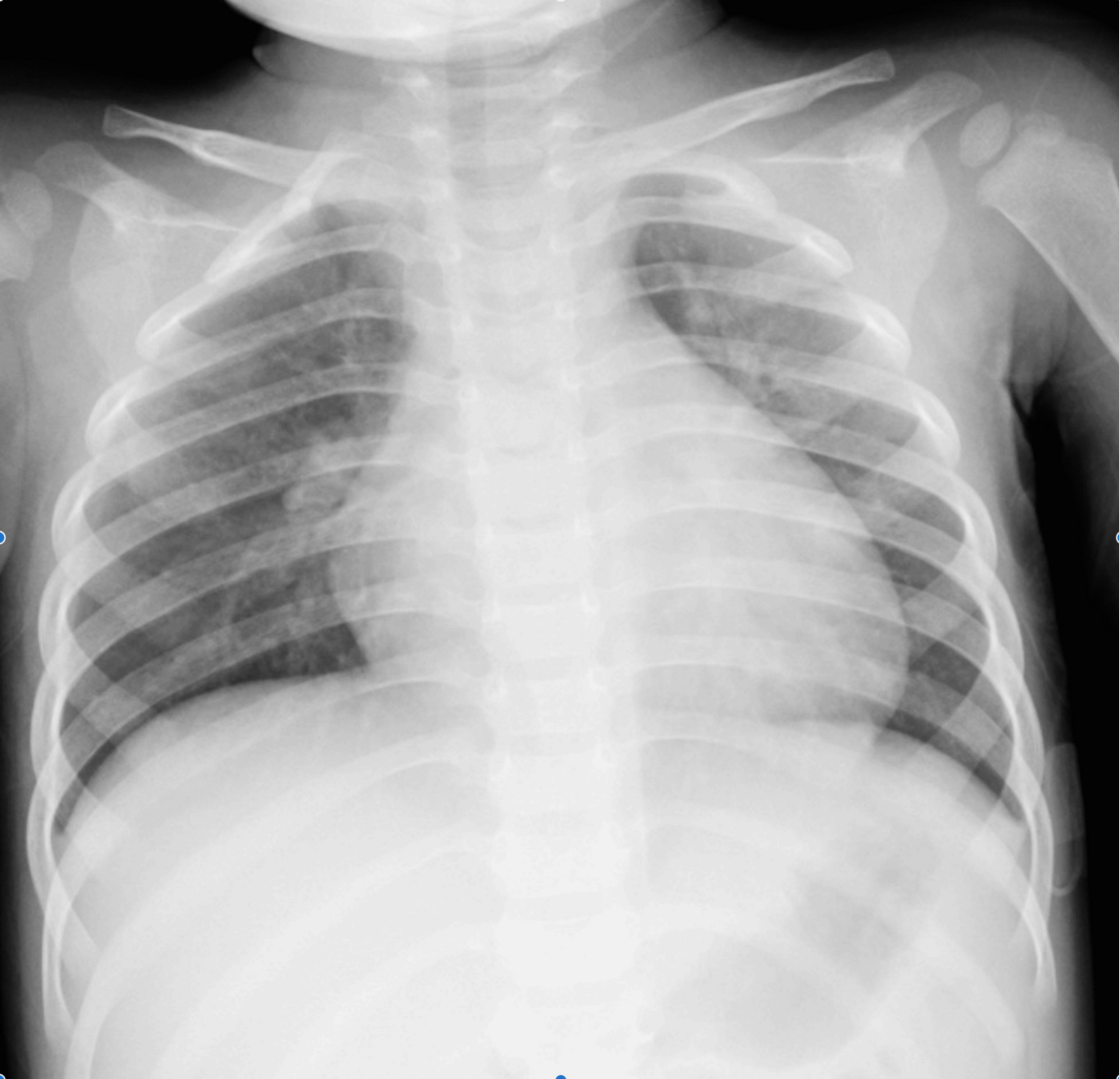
Chest radiograph on admission (cardiomegaly). Cardiomegaly with a cardiothoracic ratio of 58% was observed on the chest radiograph at admission.
